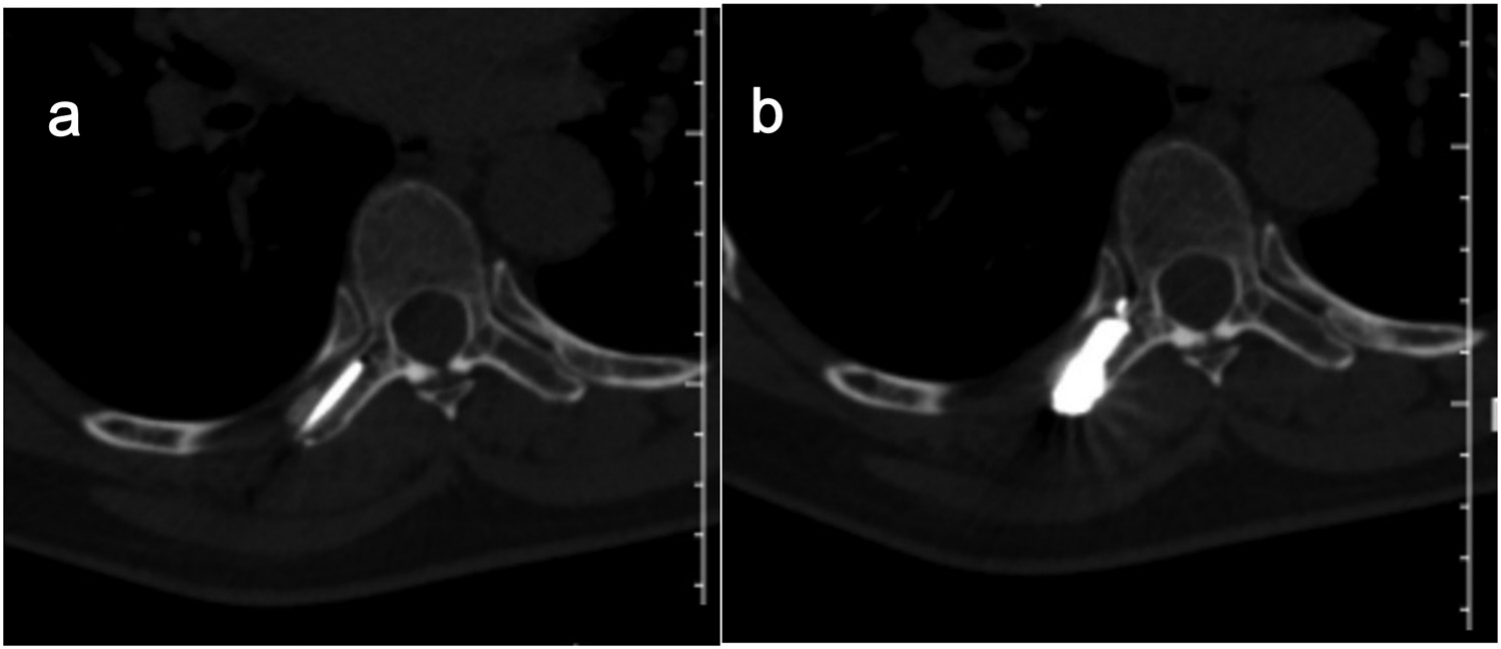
On control CT scan performed during MWA (a) and bone cement was injected into the lesion during the operation (b).
